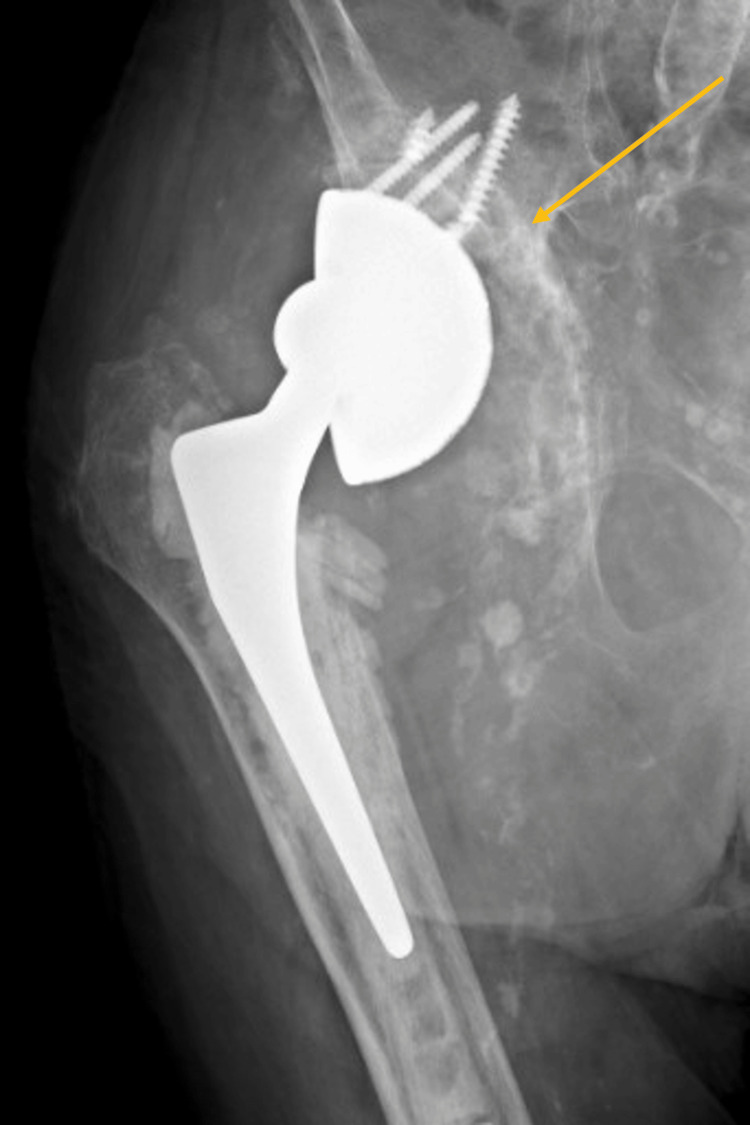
Anteroposterior pelvic X-ray showing early loosening of the revised acetabular component.
